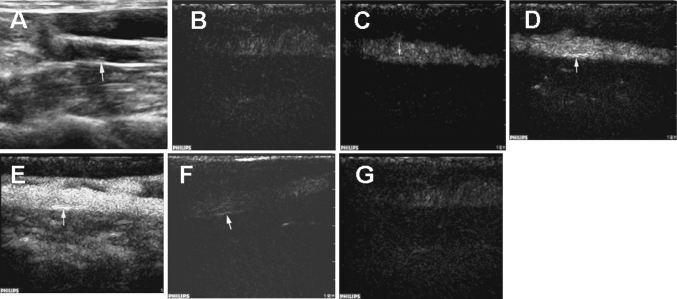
Sequential ultrasound images of IL-8-targeted imaging for an atherosclerotic plaque of the rabbit abdominal aorta. a Conventional two-dimensional view. b–g Sequential targeted CEU view: b 3.8 s post-contrast agent injection, c: 4.3 s post-contrast agent injection, d 4.9 s post-contrast agent injection; e 9.8 s post-contrast agent injection, f 68 s post-contrast agent injection, g 176 s post-contrast agent injection
